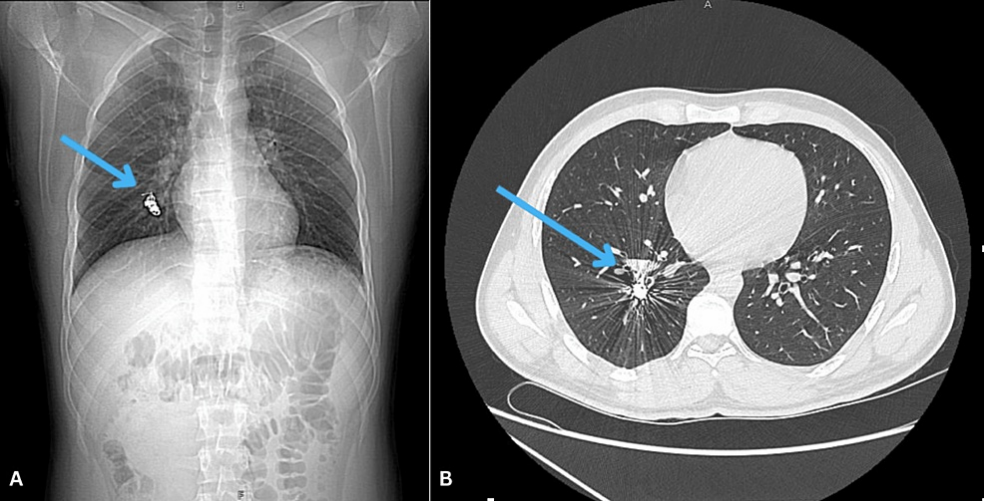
A) Post microcoil embolization. Pulmonary arteries (PA) chest and abdomen X-ray, with blue arrow showing the multiple micro coils in the aneurysm; B) Chest CT with arrow showing the micro coils
